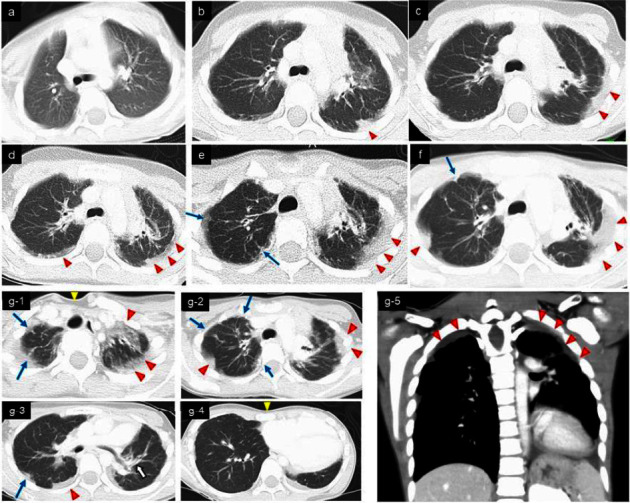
Chest CT images show progressive pleural and pulmonary fibrosis/scarring consistent with PPFE: ((a) 2015 (at the time of discharge), (b) 2017, (c) 2018, (d) 2019, (e) 2020, (f) 2021, and (g) 2022 (at the age of 9 years)). CT image shows pleural thickening (red arrow heads) and bronchiectasis (white arrow), a reticular shadow (blue arrows) localized in the bilateral upper lobes predominant. Pectus excavatum (yellow arrows) is also observed.
